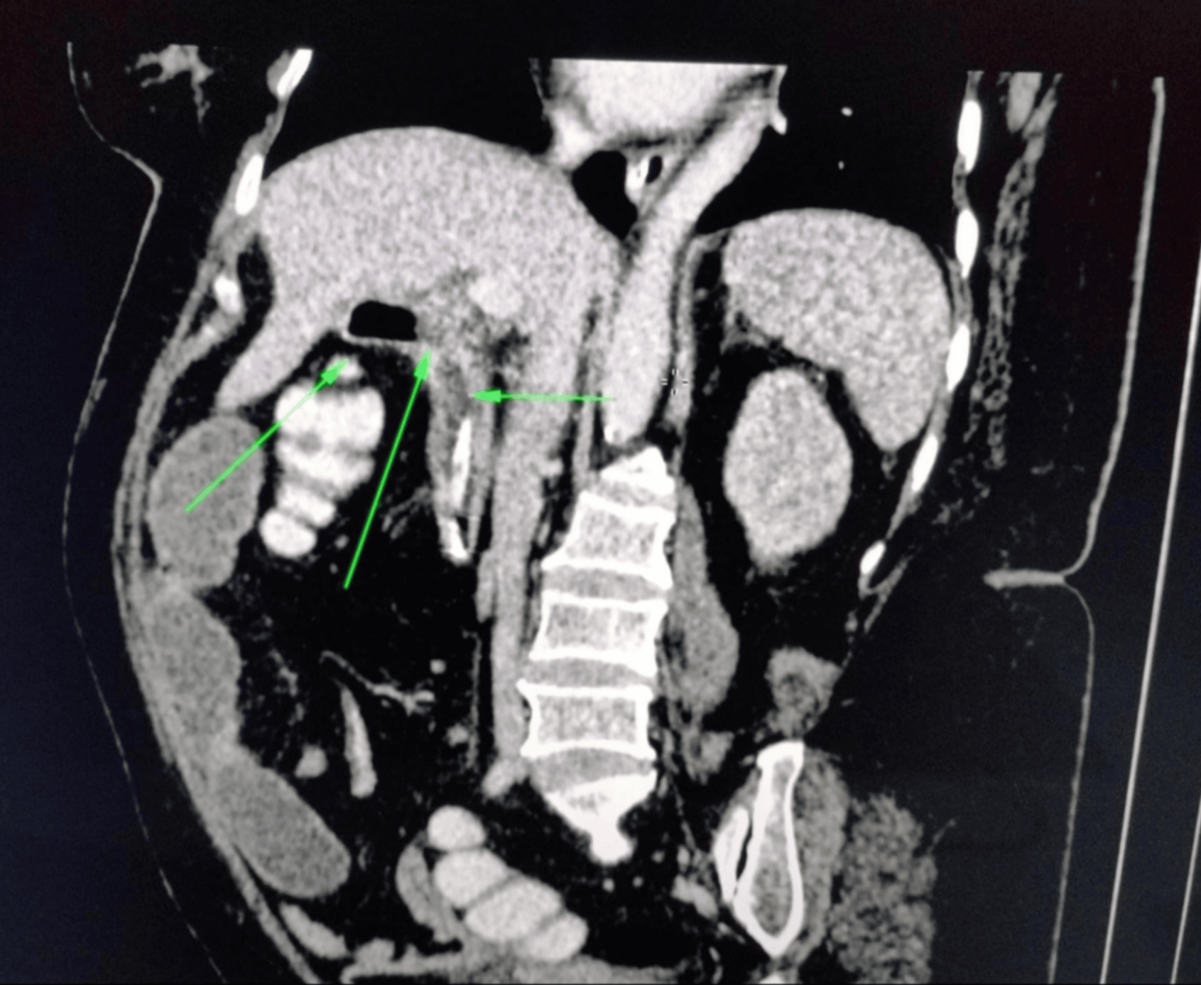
Coronal computed tomography (CT) scan demonstrating a fistulous tract between the gallbladder and colon (arrows), consistent with a cholecystocolonic fistula.
